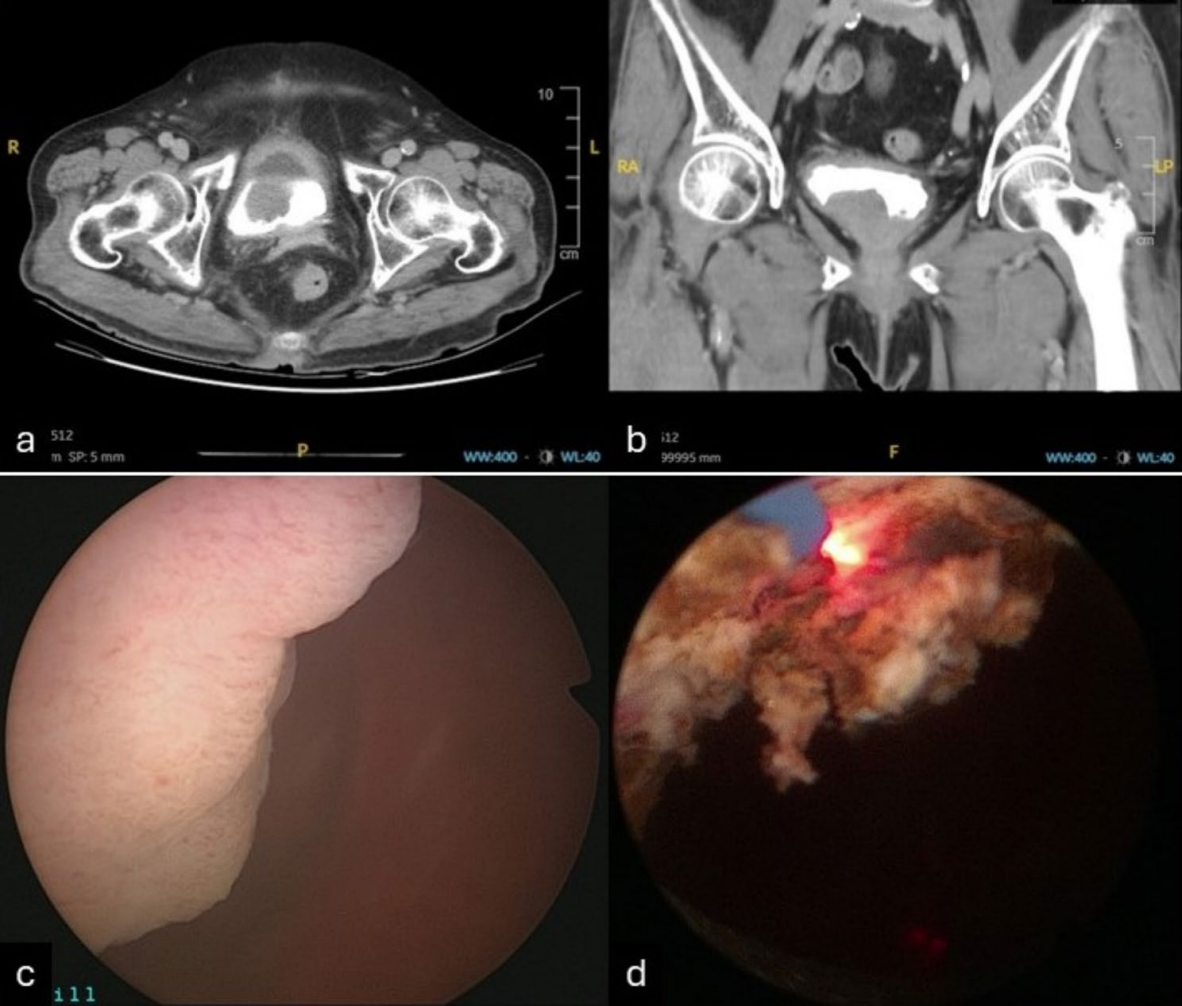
(a-b) The CT revealed a 3.9 cm mass on the anterior wall of the bladder. (c-d) During TURBT, the bladder tumors on the anterior wall near bladder neck were resected using a thulium laser under an Fr. 27 transurethral endoscope
